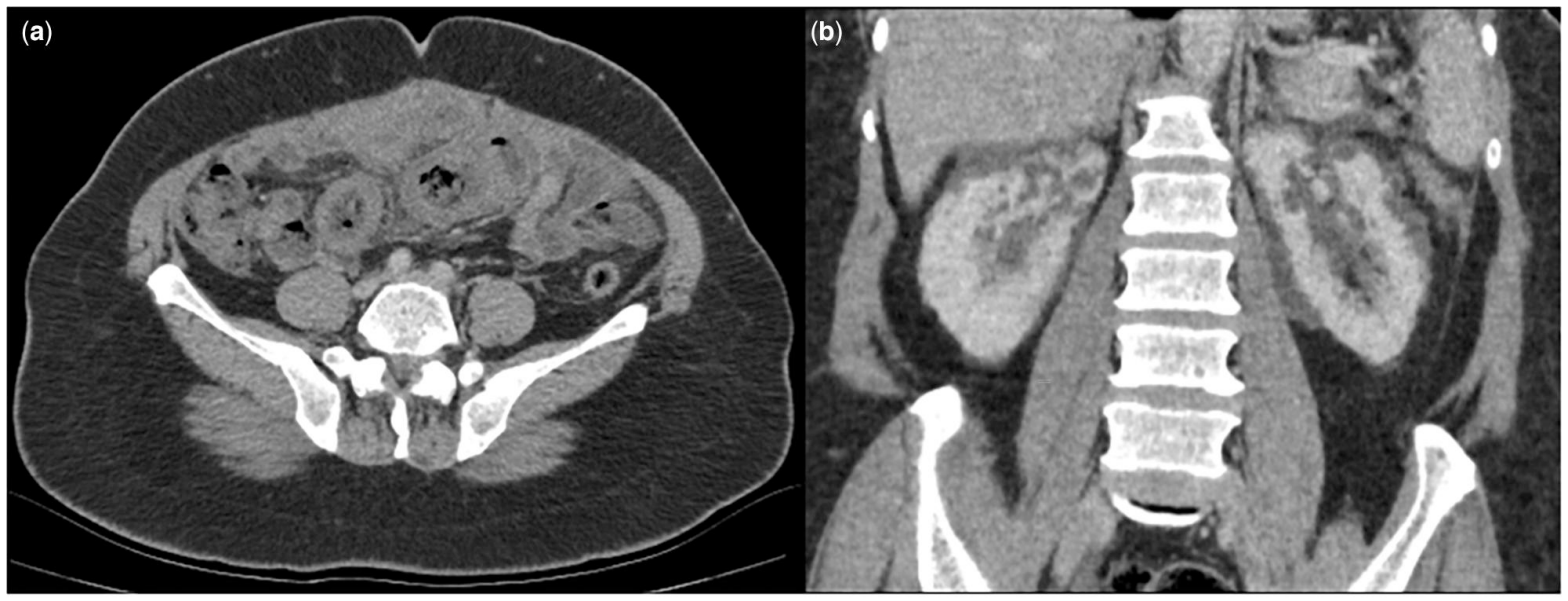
Axial and coronal contrast-enhanced abdominal CT scan demonstrates diffuse symmetric wall thickness increase in the small intestine (A) with bilateral perirenal and intrarenal cystic lesions (B).
